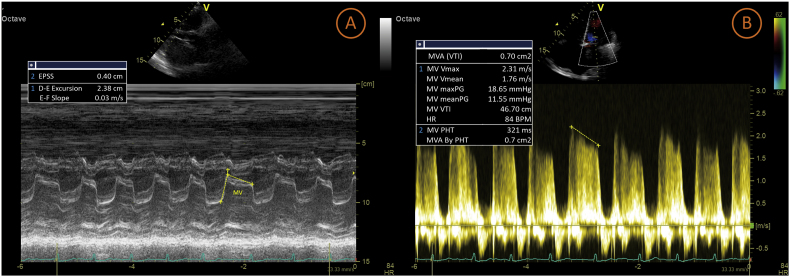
(A) The mitral valve shows slow early diastolic closure on an M-mode echocardiographic examination. The E-F slope, or mid-diastolic closure velocity, is significantly decreased or even flat, (B) Doppler Echocardiography shows severe MS.
